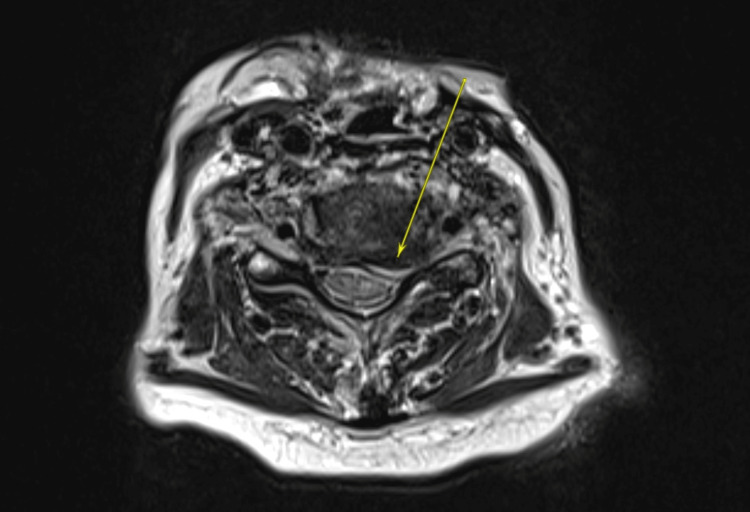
Post-contrast T2 axial MRI of the cervical spine demonstrated areas of epidural enhancement involving the ventral epidural space, including this C5-C6 level
